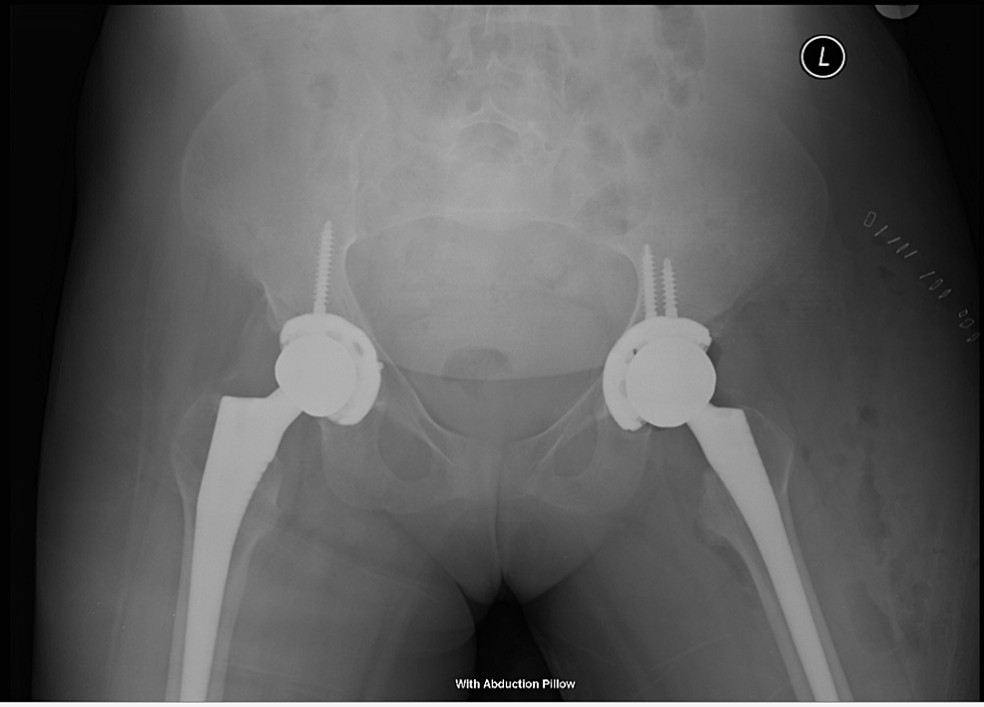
X-ray of the pelvis in 2018 after the second surgery.
